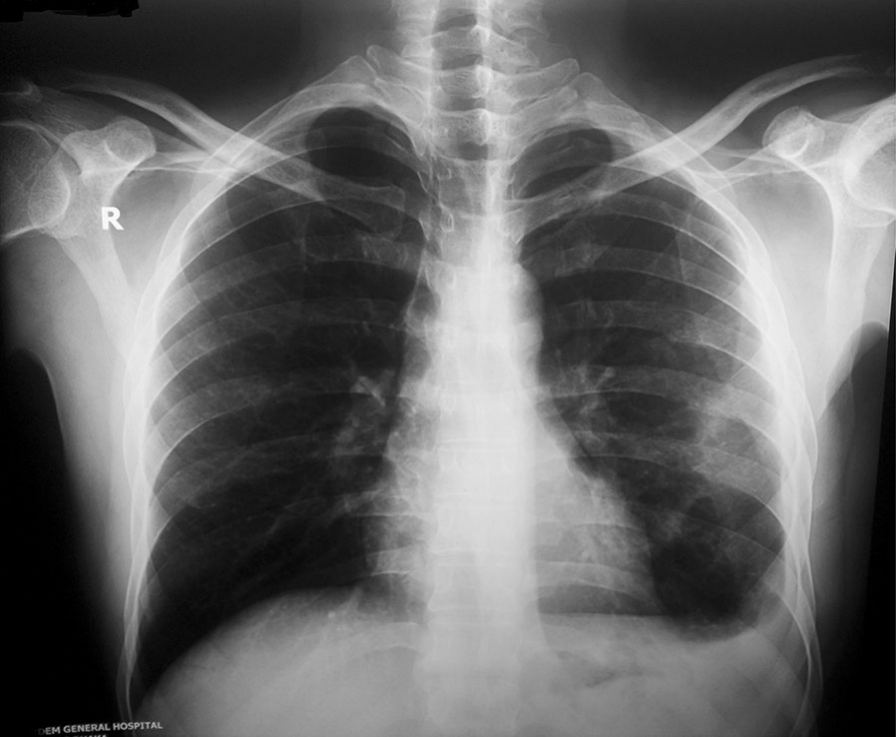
Second follow-up chest x-ray posterior–anterior view after 6 weeks of treatment showing significant radiological improvement with decreased pleural effusion (*left*)
